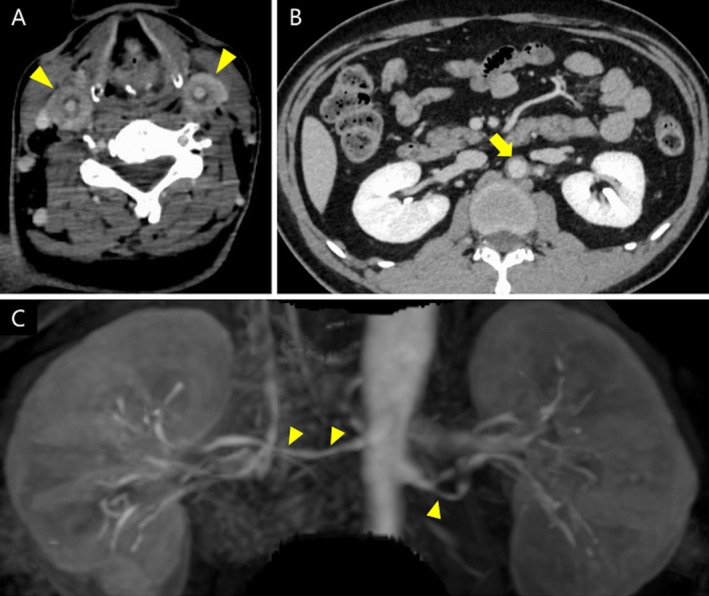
Contrast‐enhanced CT and MR imaging. (A, B) Contrast‐enhanced CT shows wall thickening with enhancement in the carotid arteries (A, yellow arrowhead) and abdominal aorta (B, yellow arrow). (C) MR angiography revealed diffuse stenosis of the bilateral renal arteries (yellow arrowhead). CT, computed tomography; MR, magnetic resonance.
